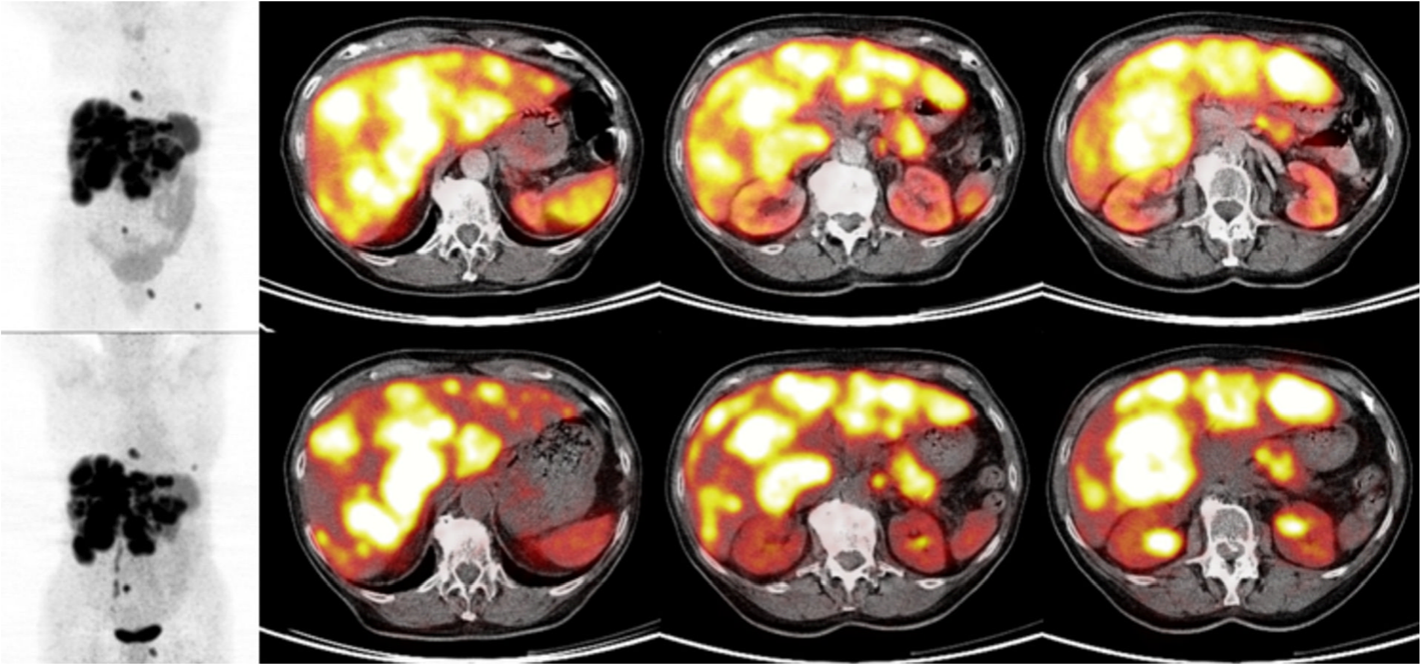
Pretreatment (above) and intra-therapeutic (below) ^68^Ga-DOTATOC PET/CT images of a patient. The patient has liver and bone metastases of a P-NET (tail of the pancreas) and high hepatic tumor disease burden. The significant increase of tumor-to-nontumor SUV ratios (SUV_T/L_ and SUV_T/S_) after ^177^Lu-octreotate administration (+111.4% and +142.2%, respectively, both *p* < 0.001) is visible on the images.
